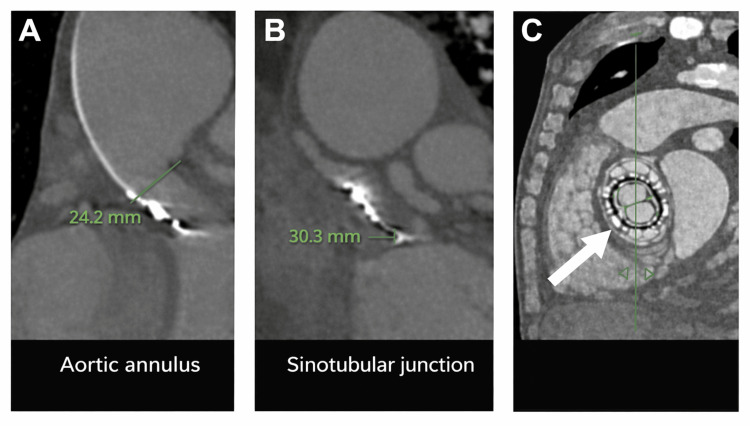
Multidetector computed tomography assessment of the aortic root. Multidetector computed tomography (MDCT) was performed using a 256-slice scanner (Philips Brilliance iCT), demonstrating measurements of the aortic root structures used for procedural planning prior to transcatheter valve implantation. (A) CT image demonstrating measurement of the aortic annulus diameter (24.2 mm) used for prosthetic valve sizing. (B) CT image demonstrating measurement of the sinotubular junction diameter (30.3 mm), an important anatomical landmark for valve positioning. (C) Multiplanar CT reconstruction demonstrating the spatial orientation and positioning of the prosthetic valve within the aortic root (arrow). These images represent original CT images obtained from the 74-year-old woman patient described in this case report, and written informed consent for publication was obtained.
